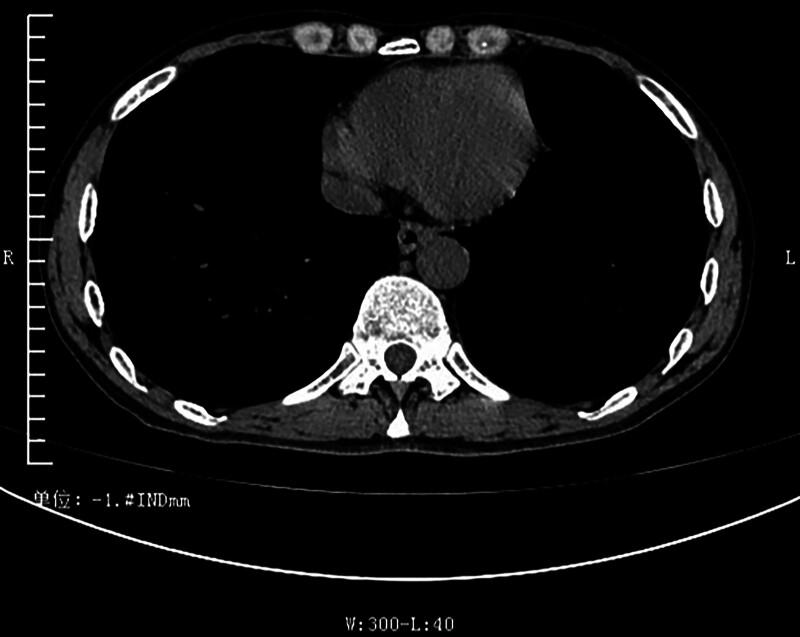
Axial mediastinal window. Follow-up chest CT at 2 months postoperatively showing no recurrence of pleural effusion.
